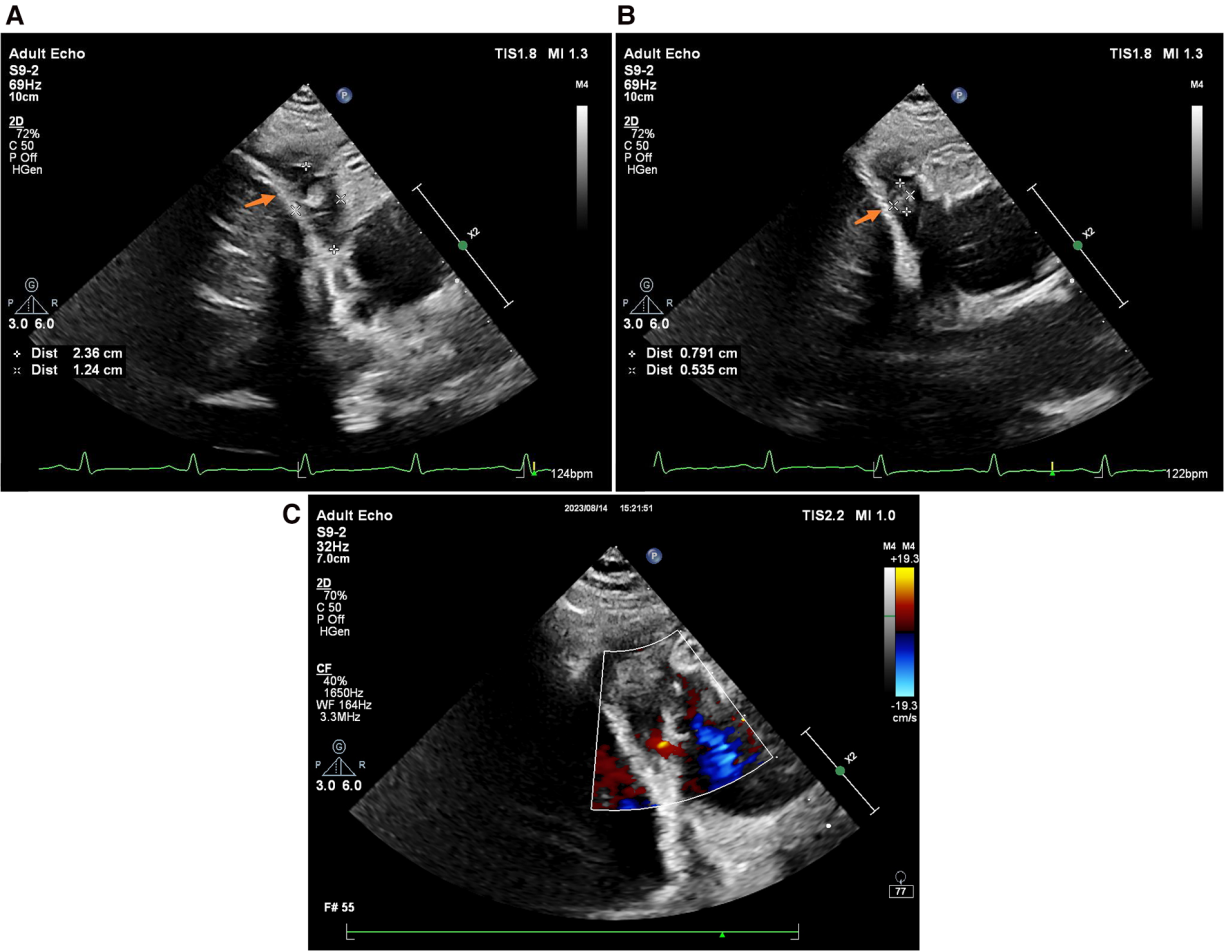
2D TTE (without pericardial effusion): (A) RAA (indicated by the orange arrow); (B) thrombus (indicated by the orange arrow); (C) color Doppler ultrasound.
